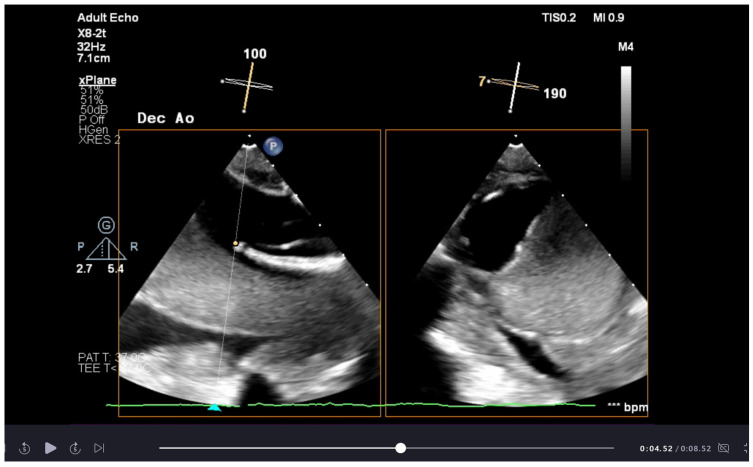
Transoesophageal echocardiogram image of thrombosis of false lumen post deployment of aortic graft.
